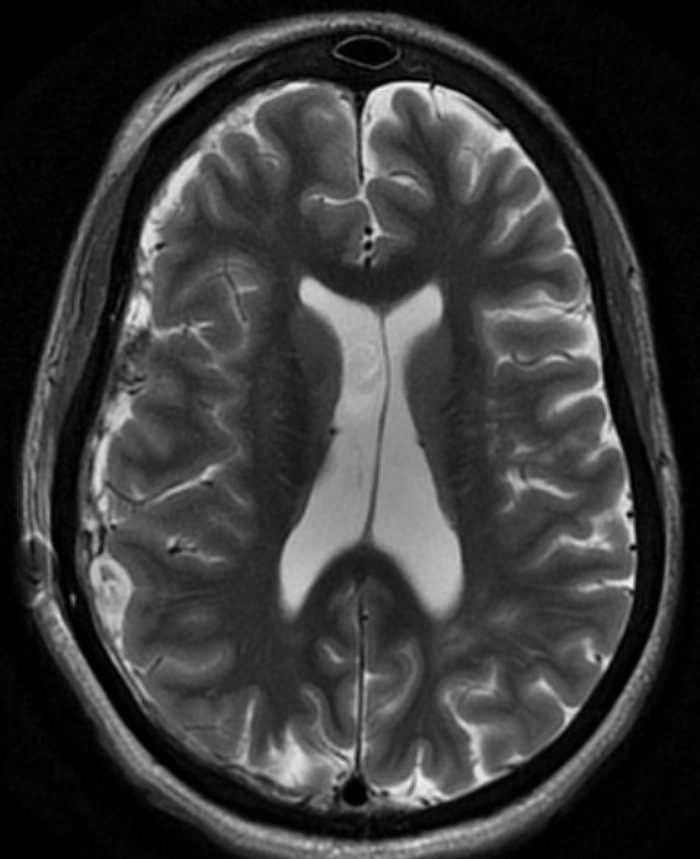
Magnetic resonance imaging of the head on admission showed right-sided subdural hematoma, 5.8-cm midline shift, and cortical laminar necrosis.
